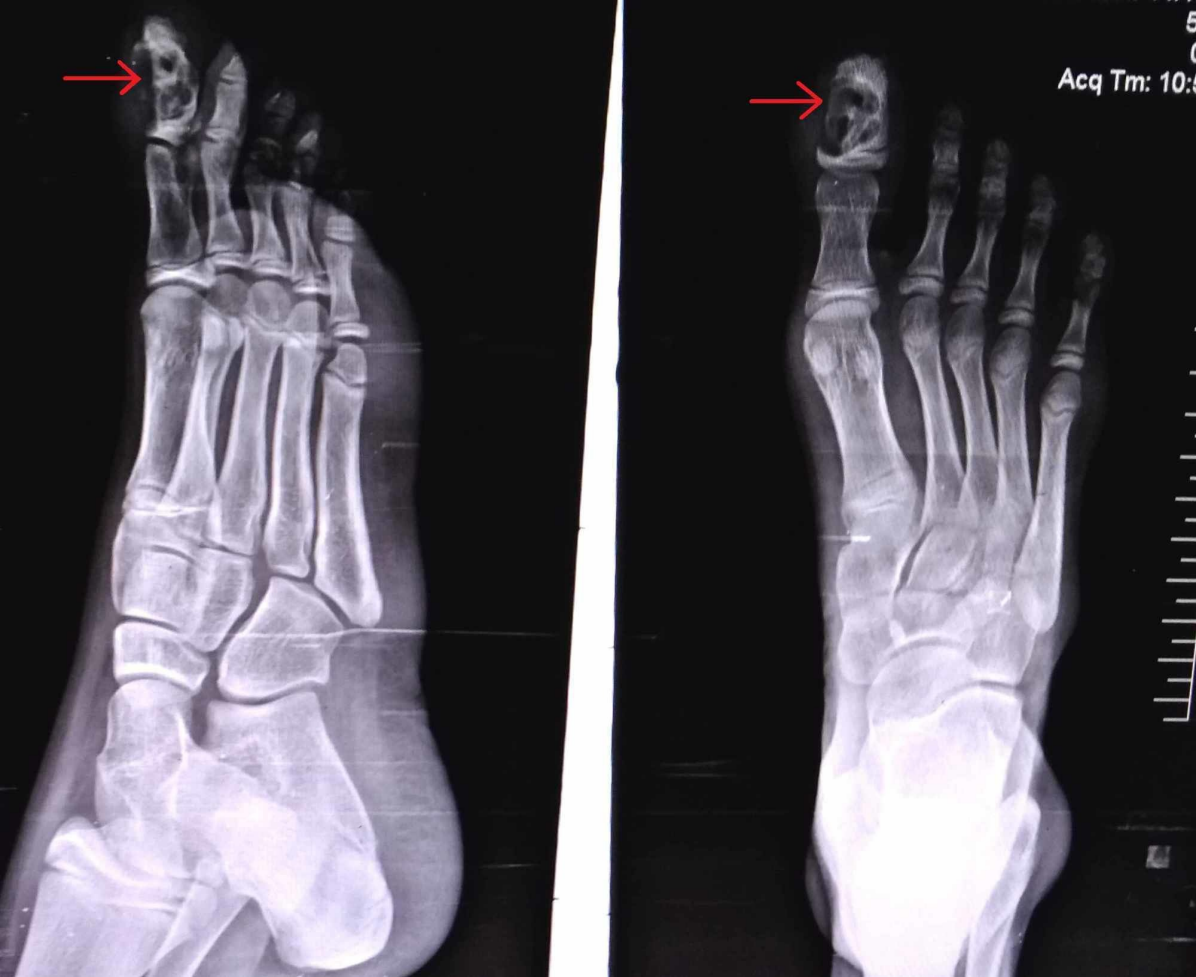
Anteroposterior and lateral view X-ray of the right foot The image shows recurrence of the tumor in the great toe
